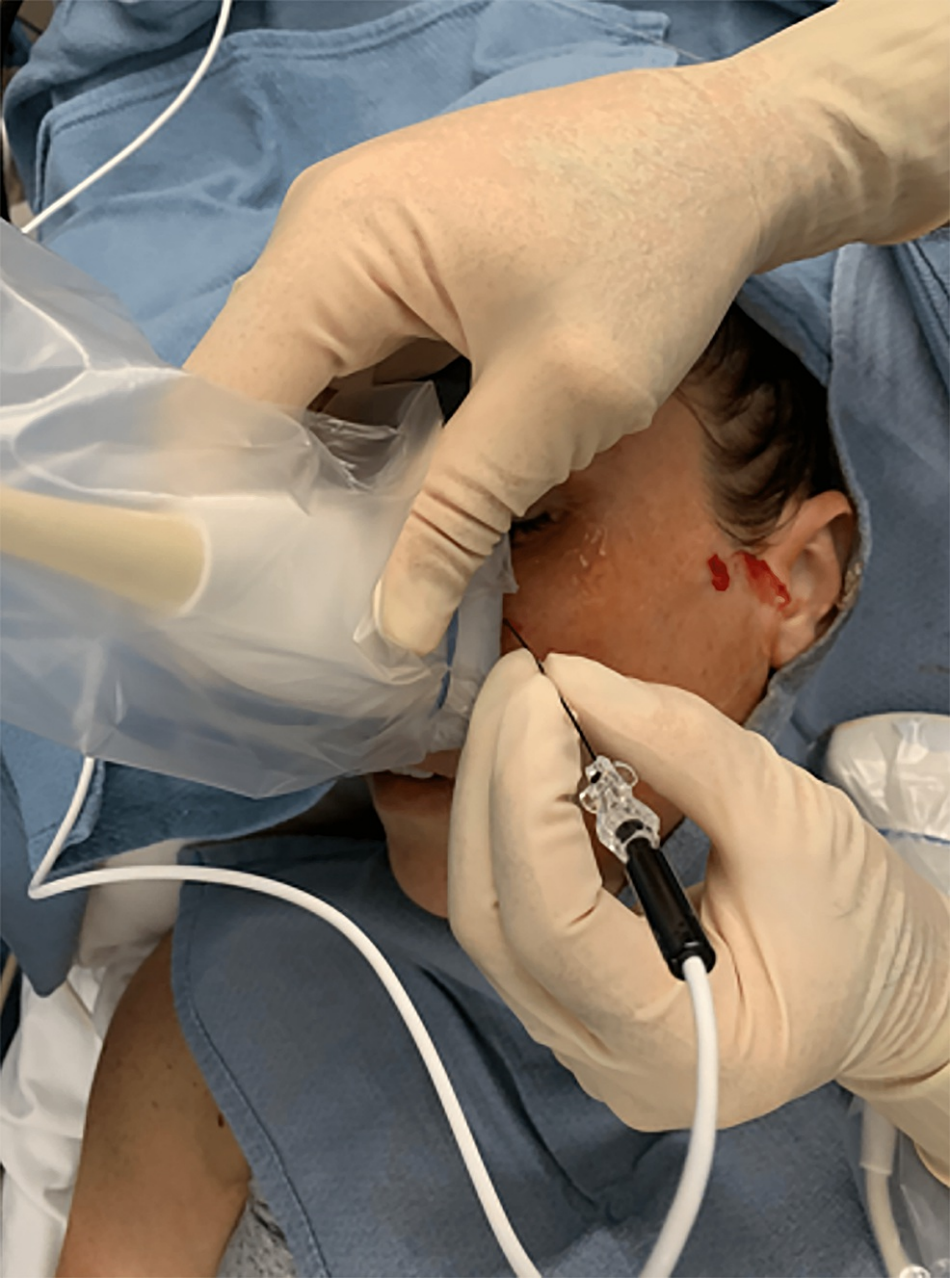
Ultrasound guided PRF of the infraorbital nerve. PRF: Pulsed radiofrequency
